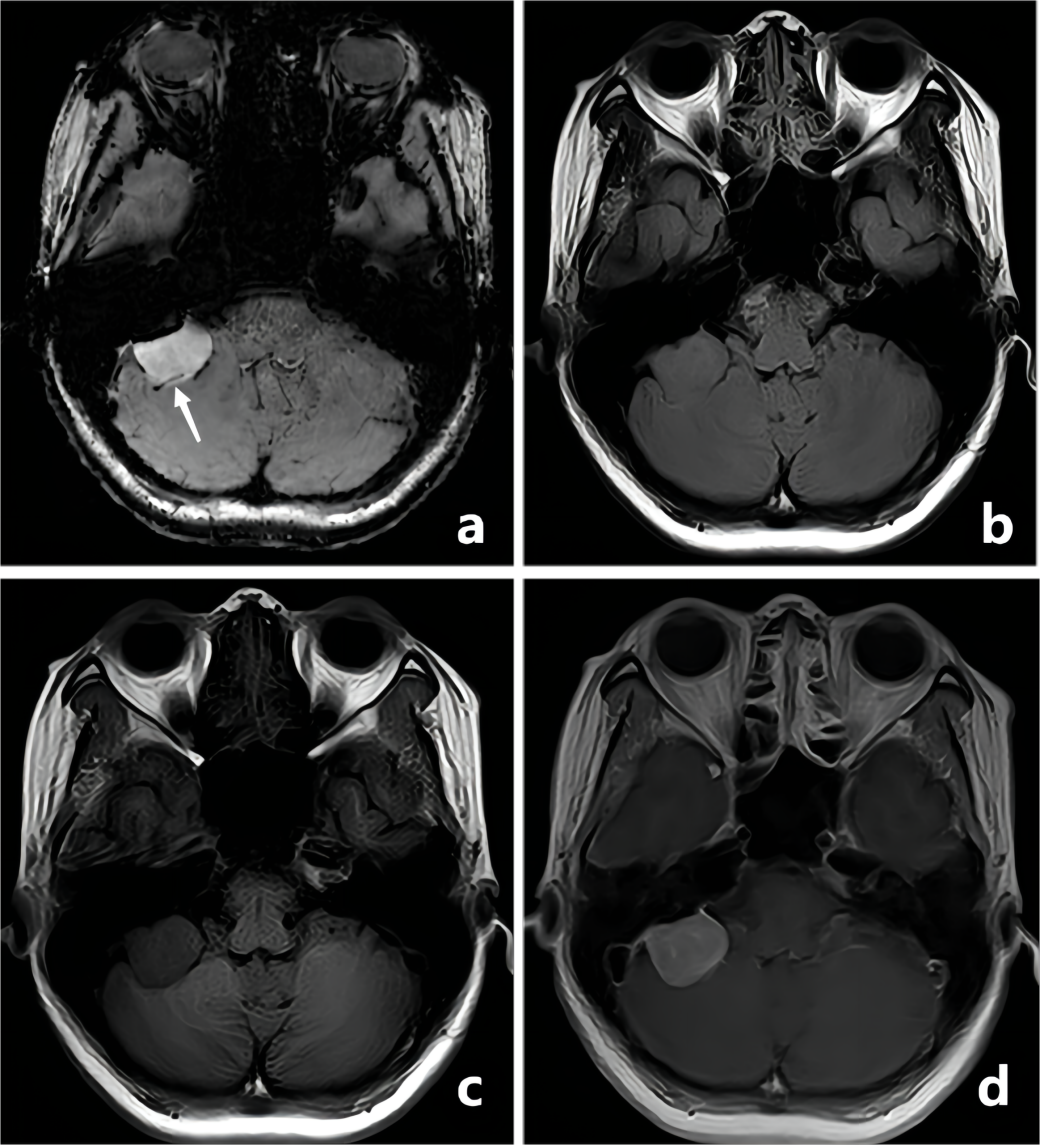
A-50-year-old woman with a pathologically confirmed meningioma in her right cerebellopontine angle area after surgical resection. The meningioma showed obvious homogeneous enhancement, and the intratumoral blood supply vessel appeared as linear hypointensity on CE-T_1_WI FSBB (A, white arrow). FLAIR (B), T_1_WI (C), and CE-T_1_WI (D) showed no signs of blood vessels.
